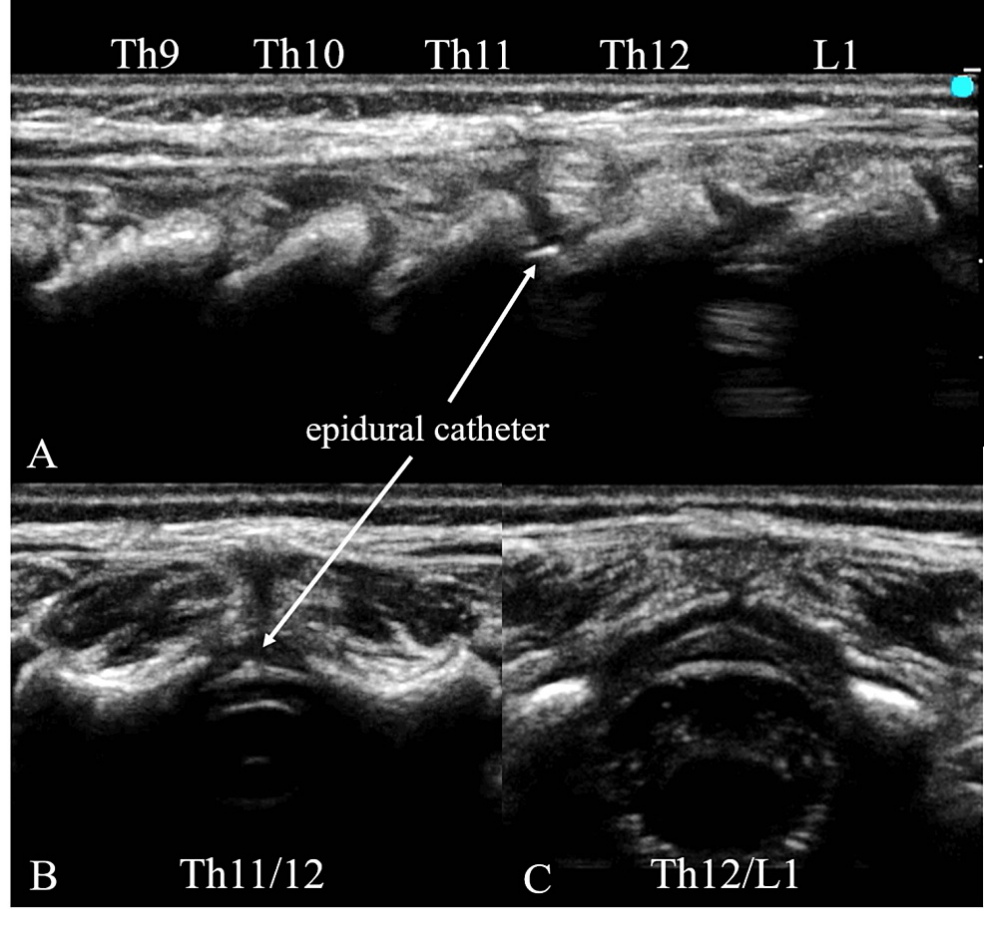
Ultrasound examination of the spine A: Sagittal view of the spine. The epidural catheter, as a hyper-echoic structure (arrow), was detected at Th11/12. B: Axial view of Th11/12. The remainder of the catheter, as a hyper-echoic dot, was detected in the epidural space. C: Axial view of Th12/L1. Nothing was detected in the epidural space.
